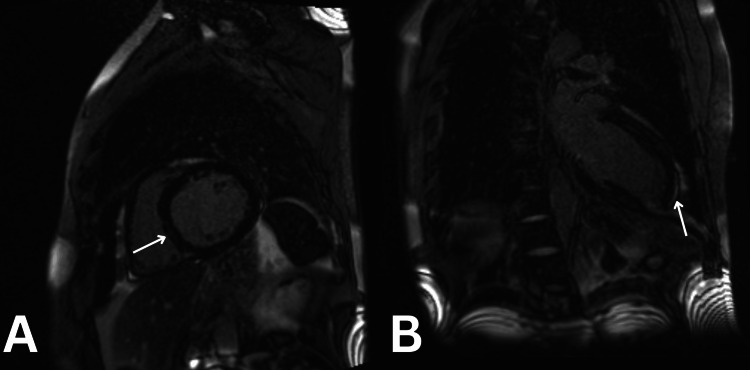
Cardiac MRI with BLG (basic late gadolinium) and thrombus sequences (perfusion and early gadolinium) technique. A. Inferoseptal transmural post-ischaemic scar (arrow); and B. apical inferior transmural post-ischaemic scar (arrow).
